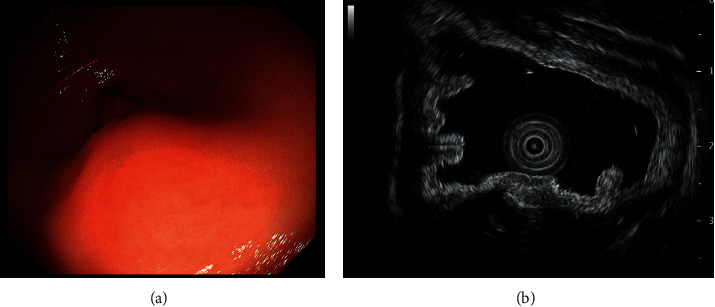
(a) Esophagogastroduodenoscopy (EGD) image. EGF revealed a pedunculated mass resembling a submucosal tumor in the antrum of the stomach. (b) Endoscopic ultrasonography image. The lesion was located in the submucosa and muscularis propria, and the mass was poorly defined and hypoechogenic.
